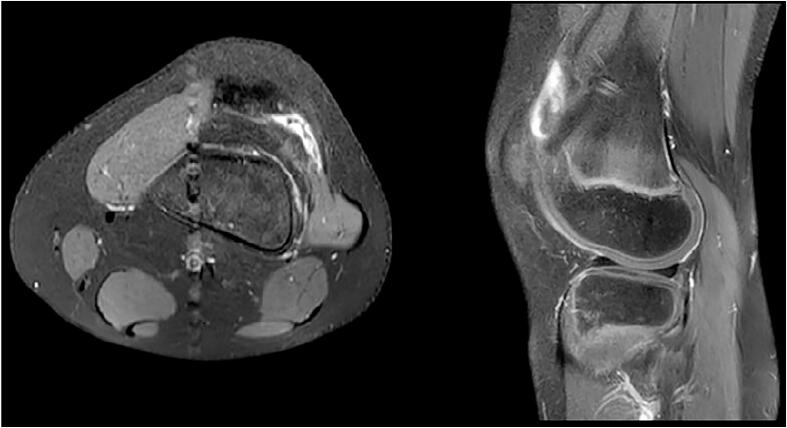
MRI of the left knee showing significant hypertrophy of the synovial membrane (a thin hypointense band lateral to the lateral femoral condyle).
